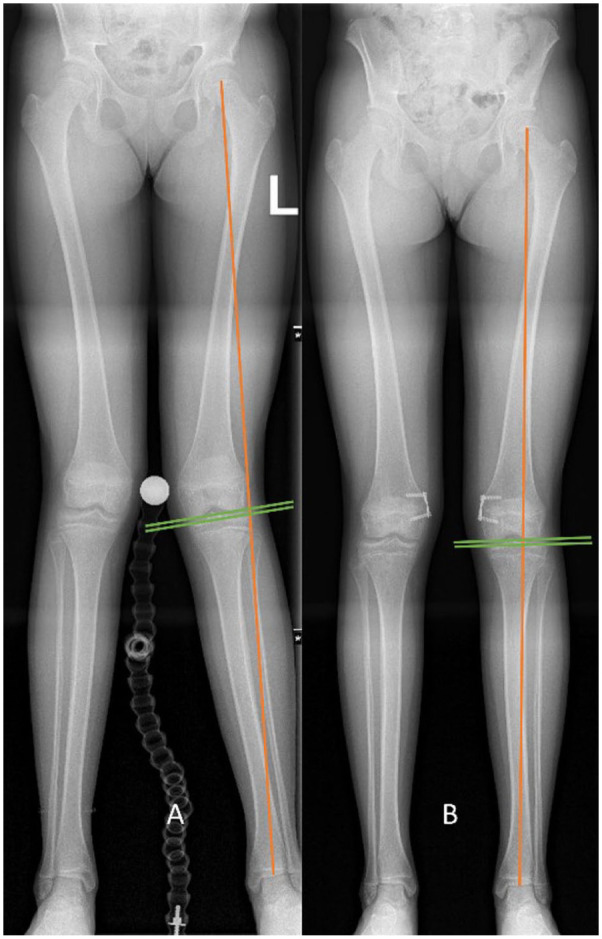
Guided growth of the distal femur to correct the mechanical axis and the genu valgum. Radiographs are preoperative (a) and after 10 month follow-up (b).
